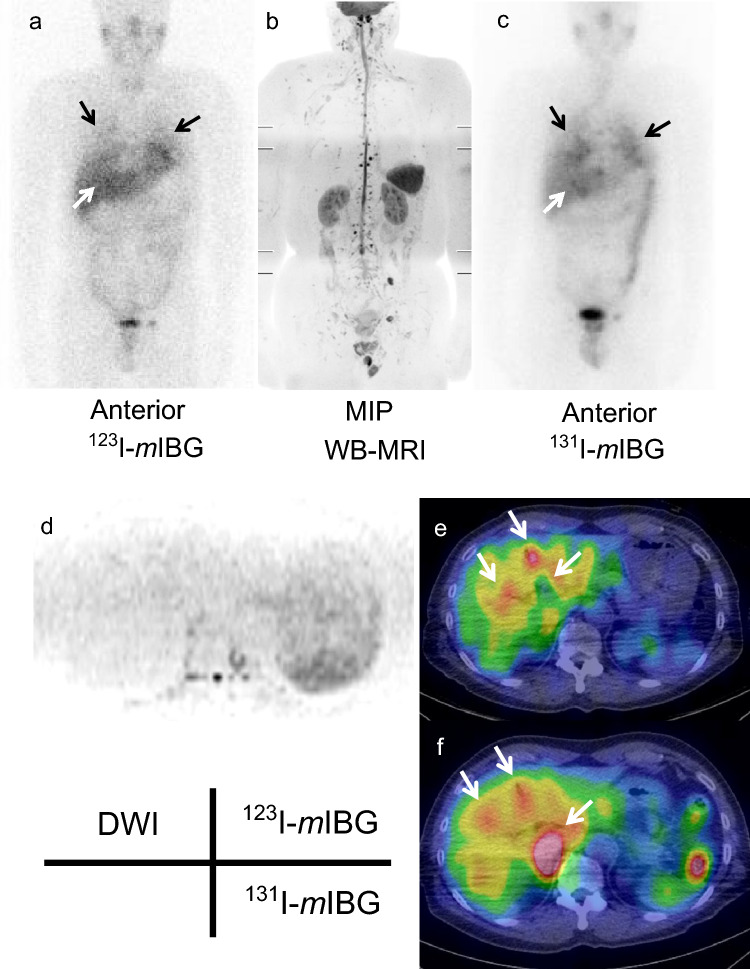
A case of multiple lung metastases with confusing liver accumulation in *m*IBG images (patient No. 7). A male patient in his 60s with multiple metastases of pheochromocytoma (lung and pelvis). Post-therapy ^131^I-*m*IBG image clearly detected multiple lung metastases (black arrows) compared with the ^123^I-*m*IBG image and WB-MRI. Conversely, *m*IBG images were confusing between liver metastases and physiologic accumulation (white arrows). (a) ^123^I-*m*IBG scintigram (anterior). (b) The MIP of WB-MRI. (c) Post-therapy ^131^I-*m*IBG scintigram (anterior). (d) DWI (axial). (e) ^123^I-*m*IBG SPECT/CT (axial). (f) Post-therapy ^131^I-*m*IBG SPECT/CT (axial). *WB-MRI* whole-body magnetic resonance image, *MIP* maximum intensity projection, *DWI* diffusion-weighted image, *T2WI* T2 weighted image, *mIBG meta*-iodo-benzylguanidine, *SPECT/CT* single-photon emission computed tomography/computed tomography.
